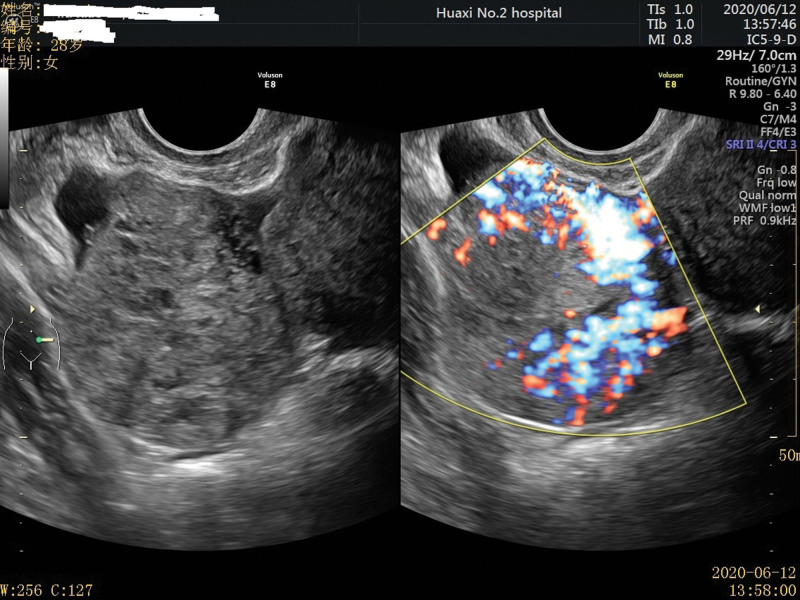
Doppler ultrasound presents the heterogeneous tumor on the left ovary with abundant blood flow signals.
